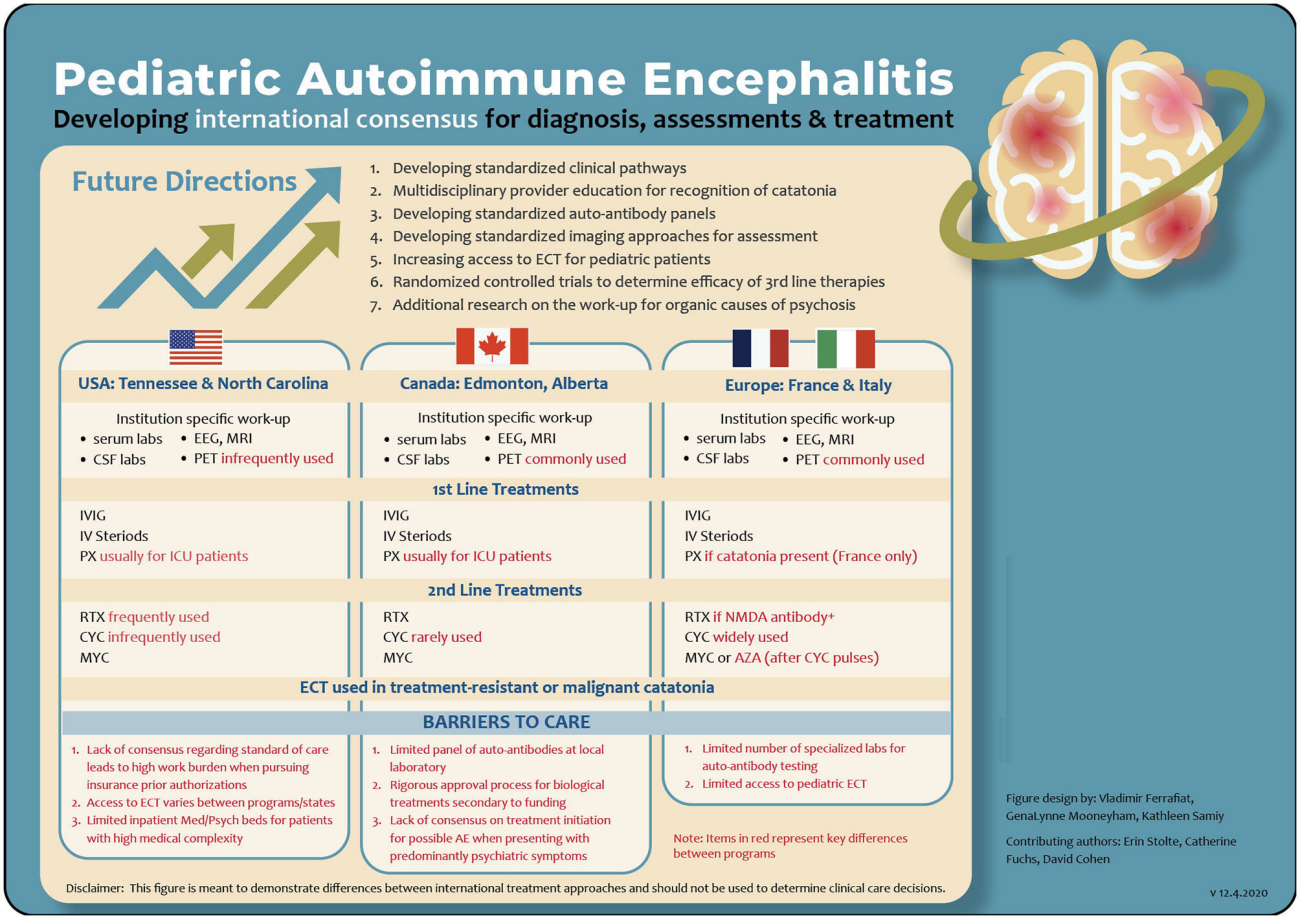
A comparison of program specific assessment and treatment pathways for AE. AE, autoimmune encephalitis; AZA, azathioprine; CSF, cerebral spinal fluid; CYC, cyclophosphamide; ECT, electroconvulsive therapy; EEG, electroencephalogram; ICU, intensive care unit; IV, intravenous methylpredinisone; IVIG, intravenous immunoglobulins; MRI, magnetic resonance imaging; MYC, mycophenolate mofetil; NMDA, N-methyl-D-asparate; PX, plasmapheresis; PET, positron emission tomography; RTX, rituximab.
